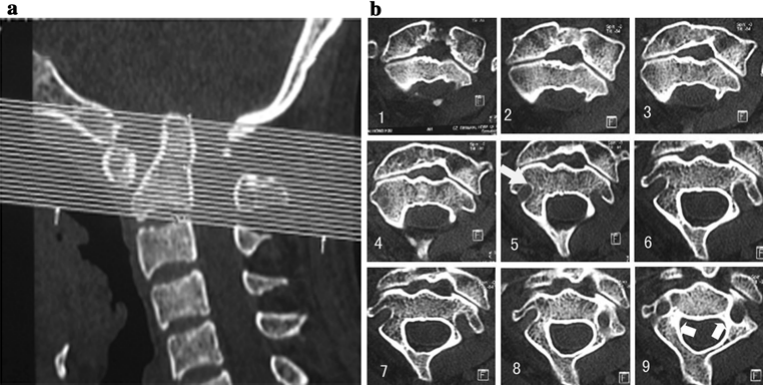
a 1-mm-thin-slice consecutive CT scan of C2 was carried out along the C2 pedicle direction (about 15°–20° superiorly). b In the consecutive axial thin-slice CT of C2, *white arrows* in the 5th slice show the apex of VGA, and then, parameter *e* could be calculated by the number of slices easily; *white arrows* in the 9th slice show the distance from the entrance of VGA to the vertebrae canal, and then, parameter *a* could be measured in this slice)
